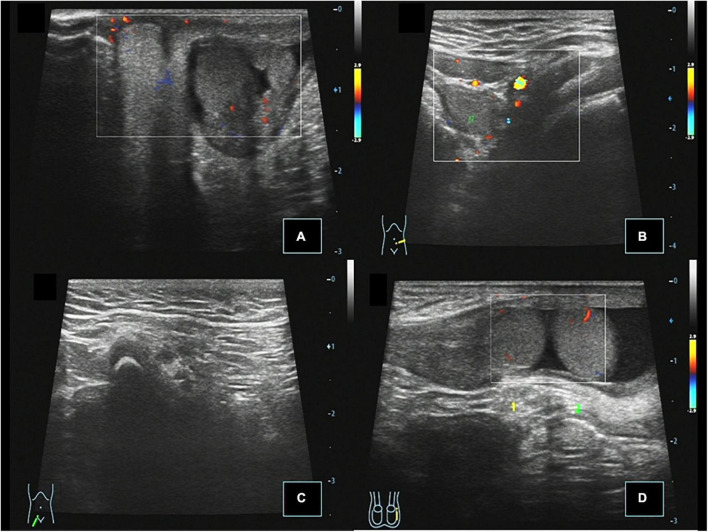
Case 14, 0.67 years, chief complaint: right scrotal emptiness. Preoperative ultrasound: (A) Cross section of the scrotum showing that the left testis is located in the left scrotum, and there was no testis echo in the right scrotum. (B) Cross section of the left lower abdomen showing that the right testis is located next to the left iliac vessel, and CDFI showed punctate blood flow signals in the testis parenchyma. (C) Longitudinal view of the right inguinal region showing no spermatic cord echo. (D) When the child was crying, the right testis passed through the left inner ring to the left inguinal canal.
